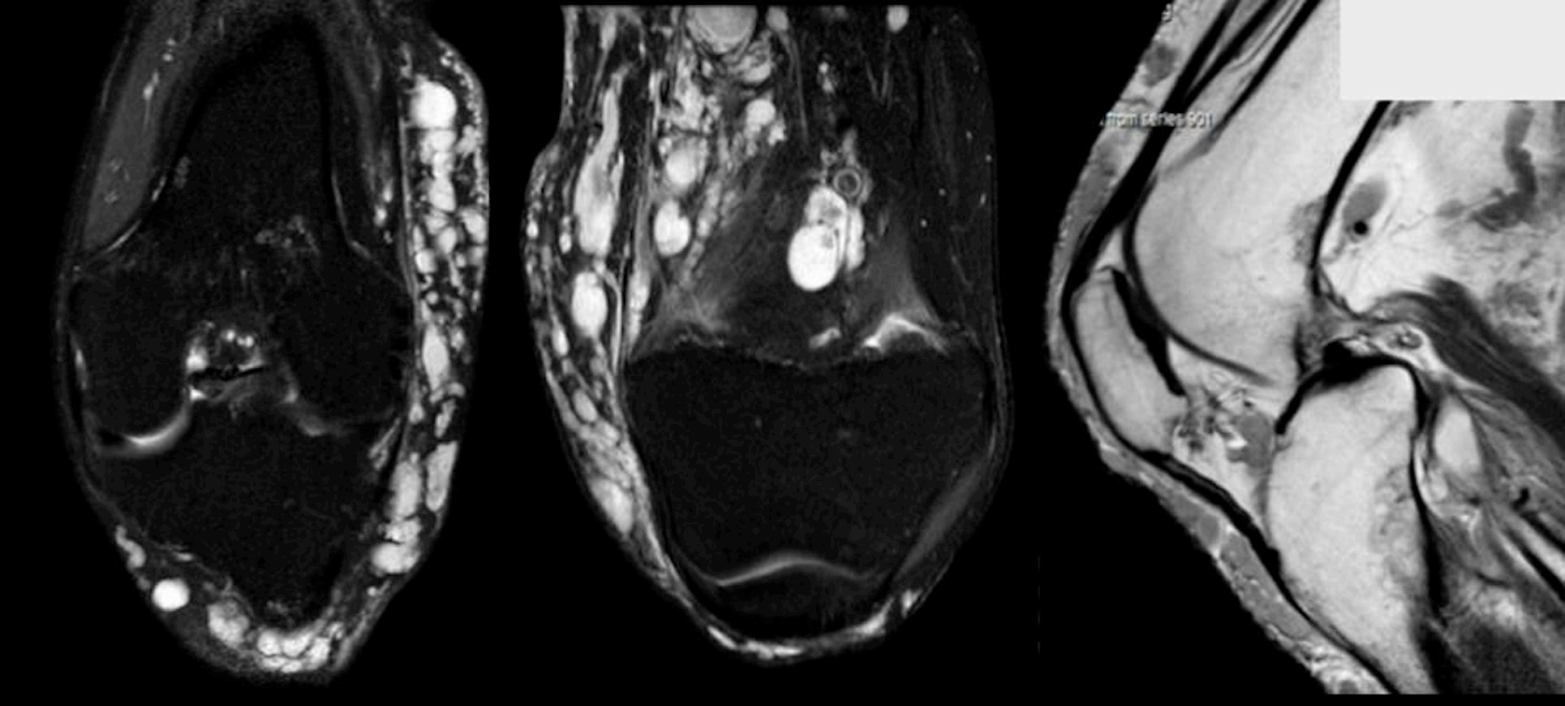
MRI shows important varicose development involving the superficial venous
